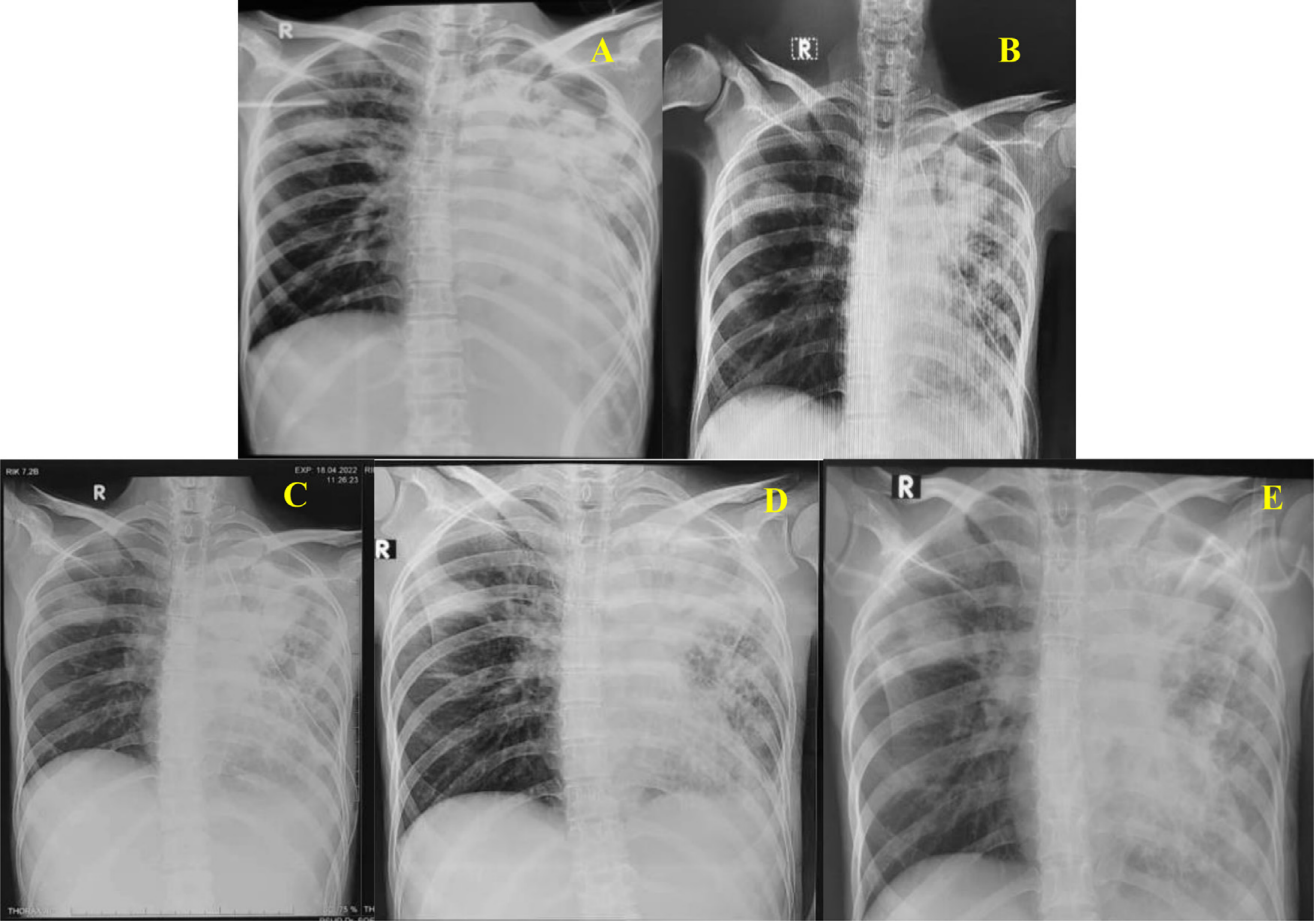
Serial chest x-ray on hospitalization (A. Day 16; B. Day 24; C. Day 26; D. Day 27; E. Day 30).
